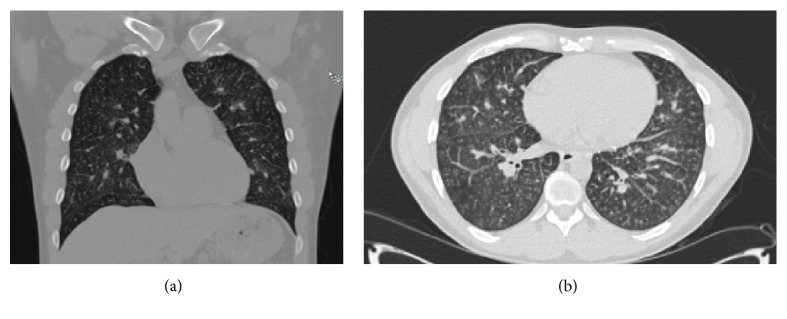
Coronal (a) and transverse (b) computed tomography views of the chest on first presentation. Images show ground-glass centrilobular micronodularities with perihilar ground-glass opacities. There is mild septal thickening and clear airways. Pulmonary artery is 3.3 cm, suggesting pulmonary hypertension.
